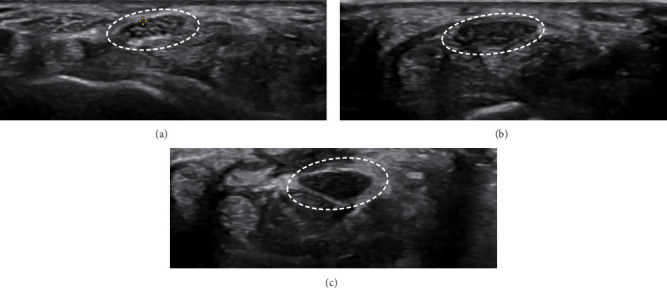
Ultrasound images of the median nerve in patients with varying severities of carpal tunnel syndrome (CTS). (a) Mild CTS, characterized by a distinct honeycomb-like pattern and preserved echogenicity. (b) Moderate CTS, showing a pronounced loss of the honeycomb pattern and further reduced echogenicity. (c) Severe CTS, exhibiting marked pattern loss and significant echogenicity reduction.
